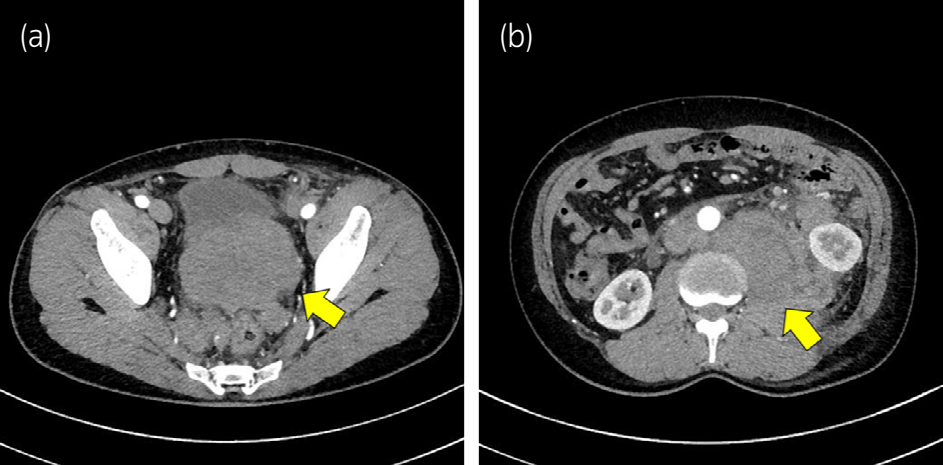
Abdominal CT findings. A huge prostate mass (a) with a volume of 220 mL extending to the left retroperitoneum (b).
